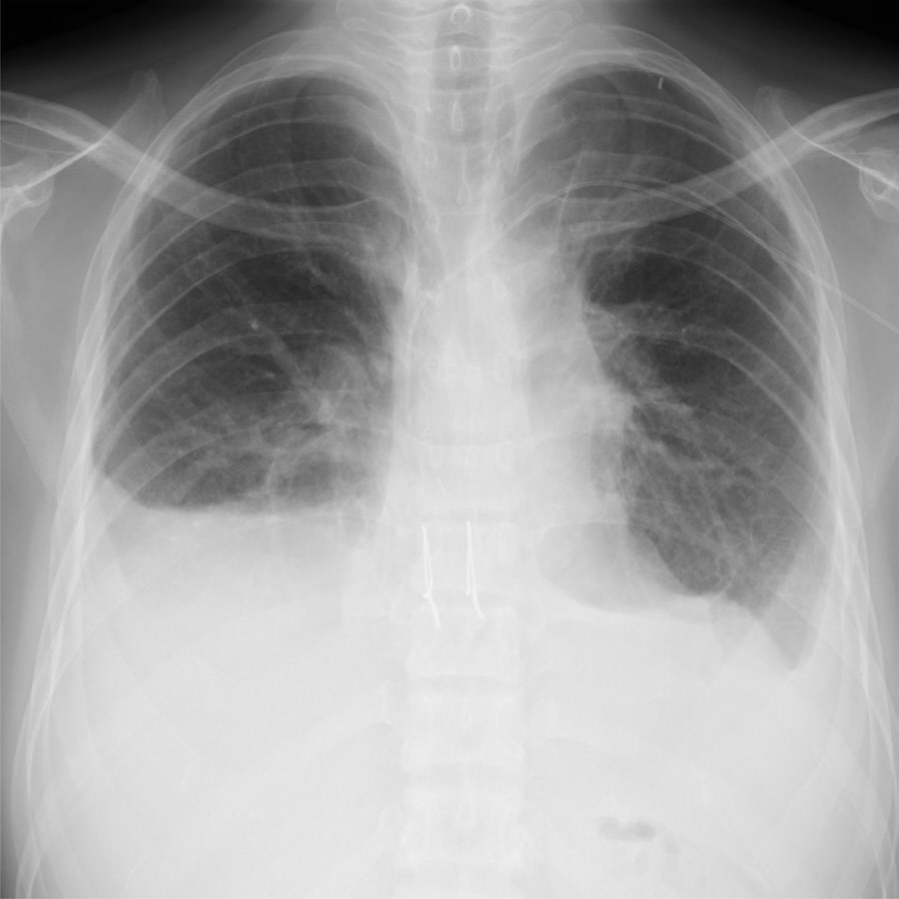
Chest radiograph before retransplantation showing pleural effusion in bilateral chest cavities
